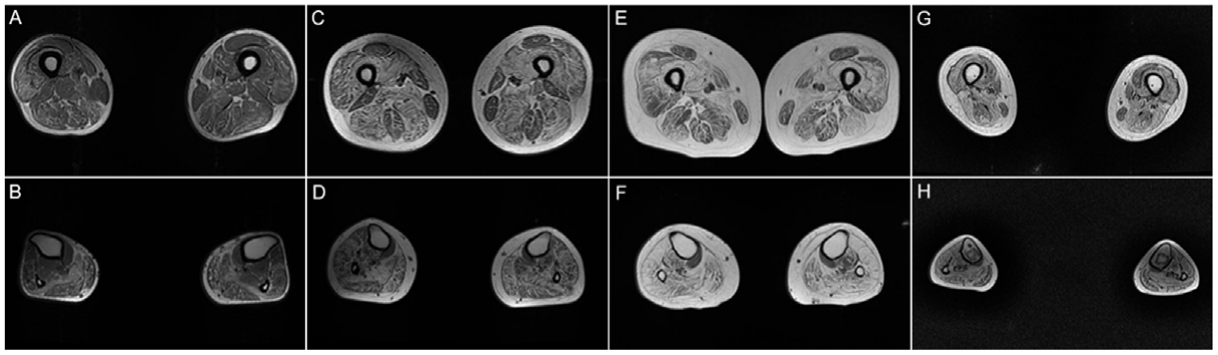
Axial T1-weighted MRI images for patients with different severities. (A) and (B) patient 13: mild weakness, ambulatory without support and with a disease duration of 6 years. (C) and (D) patient 12: mild weakness, ambulatory without support and with a disease duration of 4 years. (E) and (F) patient 7: moderate weakness, ambulatory without support and with a disease duration of 8 years. (G) and (H) patient 5: severe weakness, nonambulatory and with a disease duration of 9 years. In the early stages of the disease, low levels of fatty replacement appeared in the quadriceps, and the soleus exhibited serious involvement. With disease progression, severe fatty replacement was observed in both the thigh and lower limb muscles. Severe fatty replacement and atrophy developed during the later stages, although the vastus gracilis and short head of the biceps femoris were relatively spared.
